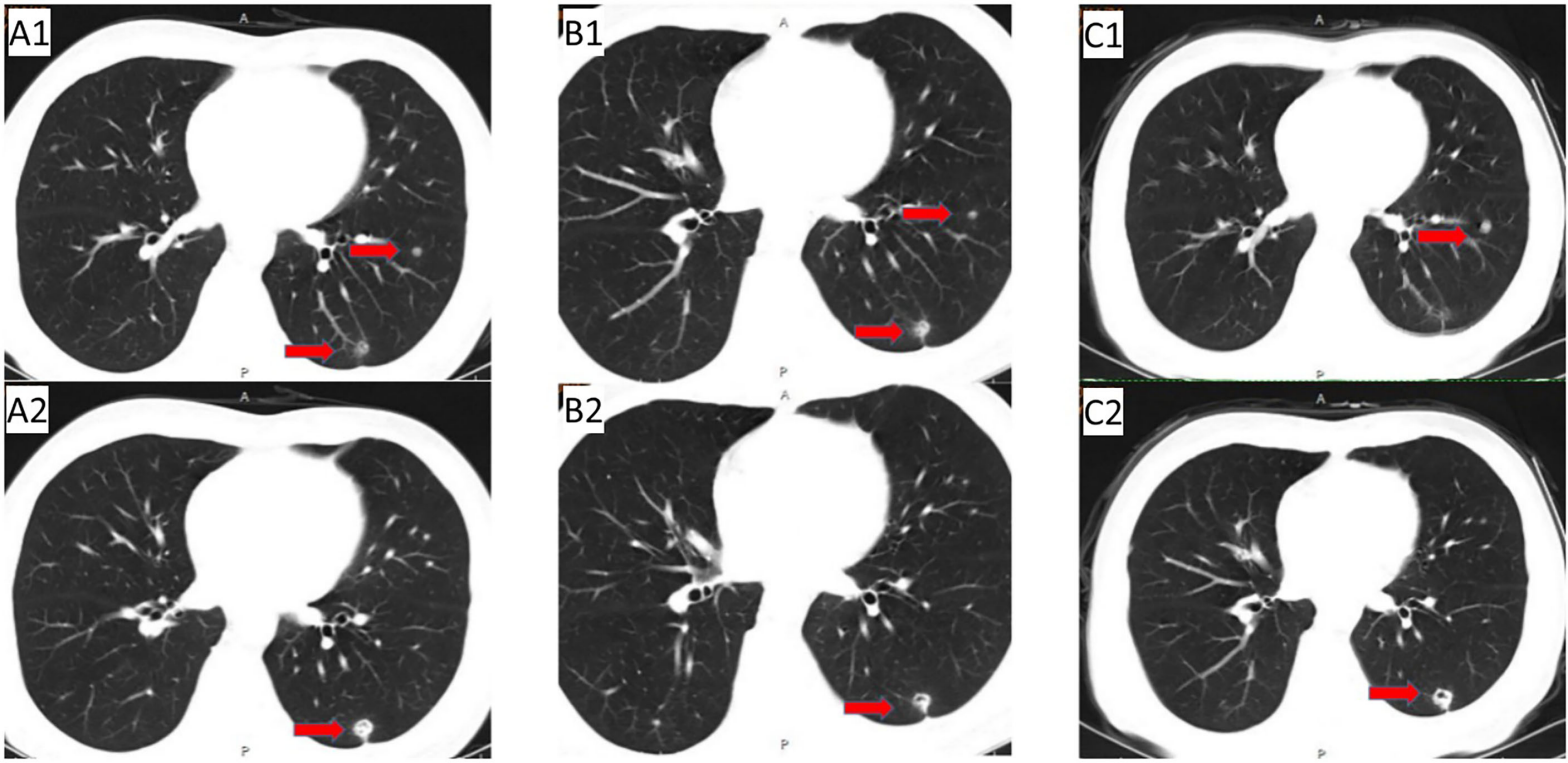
The period from the progression of lung metastasis to before the application of anlotinib + TAS-102 treatment: (A1/A2) Follow-up CT (2020.06.12): multiple nodules in bilateral lung; (B1/B2) CT (2020.08.12) after 1 cycle of second-line treatment; (C1/C2) initial application of cetuximab + TAS-102 in the third line, and discontinuation due to adverse reactions after only 2 cycles of cetuximab. On November 24, 2020, reexamination of CT showed that pulmonary nodules were increased and enlarged than before.
